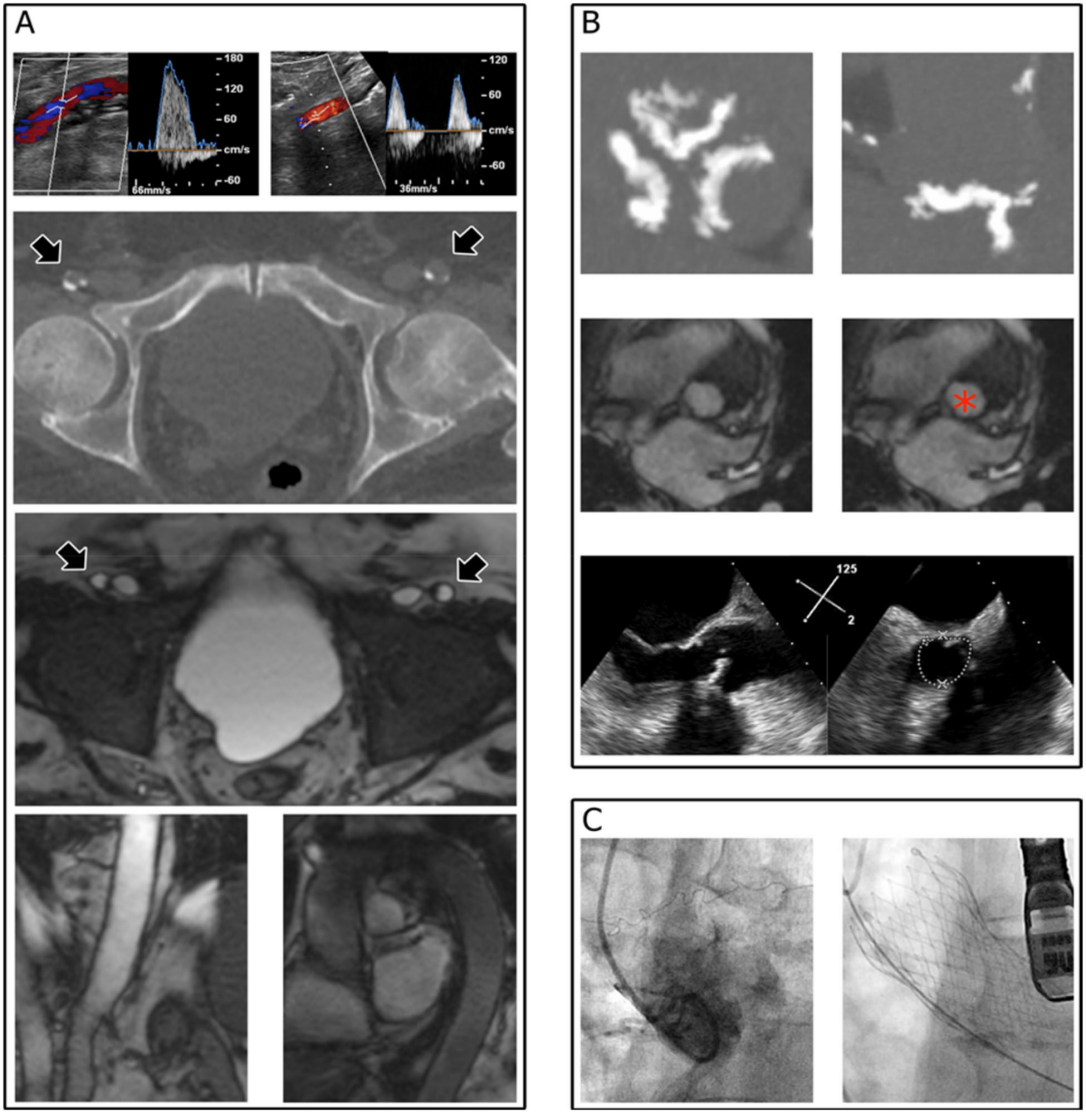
Multimodality imaging for the assessment of TAVI candidates with renal dysfunction. Femoro-iliac-aortic endovascular access (A) was evaluated by duplex scan ultrasound (upper panel showing the left and right femoral arteries), computed tomography (arrowheads in the mid-upper panel indicate the left and right femoral arteries), and nuclear magnetic resonance imaging (arrowheads in the mid-upper panel indicate the left and right femoral arteries). The aortic root and aortic annulus (B) were evaluated using computed tomography (upper panel), nuclear magnetic resonance imaging (red asterisk in the middle panel indicates the aortic annulus region), and echocardiography (lower panel), which were reassessed for confirmation during the procedure (C, left panel). The final shape of the metallic frame of the prosthesis is shown in the right panel of panel C.
